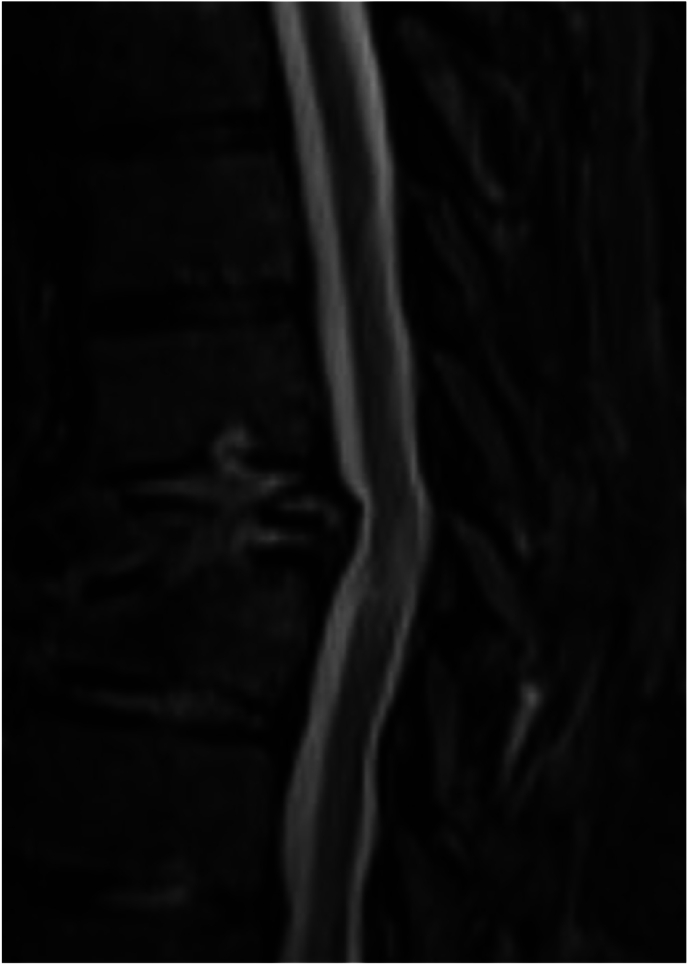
Six-month follow-up MRI thoracic spine shows a further collapse of T8 resulting in vertebral plana with new anterior aspect T9 compression fracture. Overall, improved central canal stenosis and cord signal changes with remains of retropulsed bone at T8.
